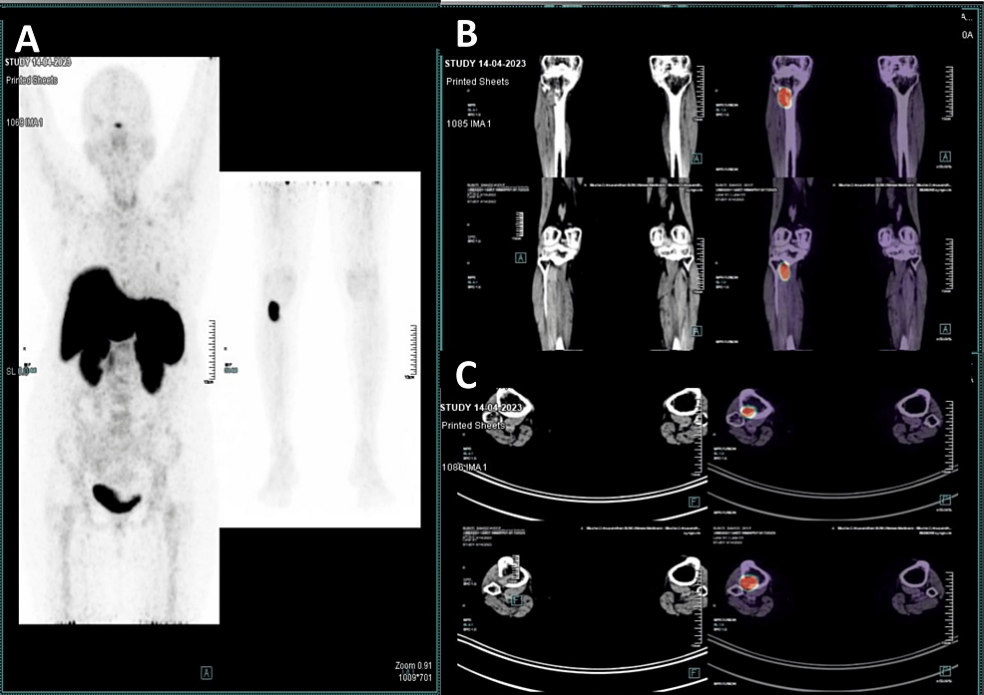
A 68-Gallium DOTANOC PET-CT scan (panel A) showing a focal increased tracer uptake in the metaphyseal aspect of the proximal right tibia (approximate size: 2.1 cm x 2.4 cm x 2.9 cm, standardized uptake values (SUV) max: 23.1). No pathological focal tracer uptake or lytic/sclerotic lesion was noted in the rest of the visualized skeleton. Panels B and C are transverse and coronal views, respectively.
